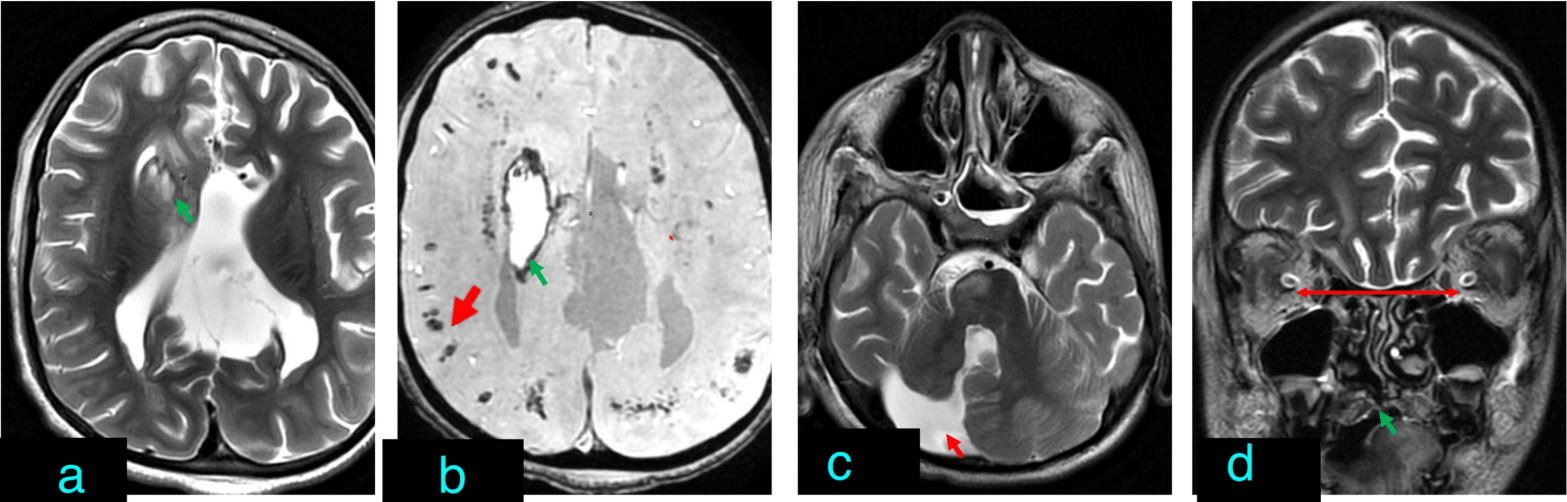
Syndromic association of corpus callosum agenesis with fronto nasal dysplasia, hypertelorism, retrocerebellar cyst and distorted brain stem (a) Axial T2-weighted imaging (T2WI) showing complete agenesis of the corpus callosum and signal alteration in the right-sided deep gray matter (green arrow). (b) Axial T2* (gradient echo) image shows areas of hemorrhage in the subcortical white matter (red arrow) as well as in the deep white and gray matter (green arrow). (c) Axial T2WI showing posterior fossa T2 hyperintense retrocerebellar cyst connecting with the 4th ventricle (red arrow). (d) Coronal T2WI showing cleft palate (green arrow) and hypertelorism (red line).
